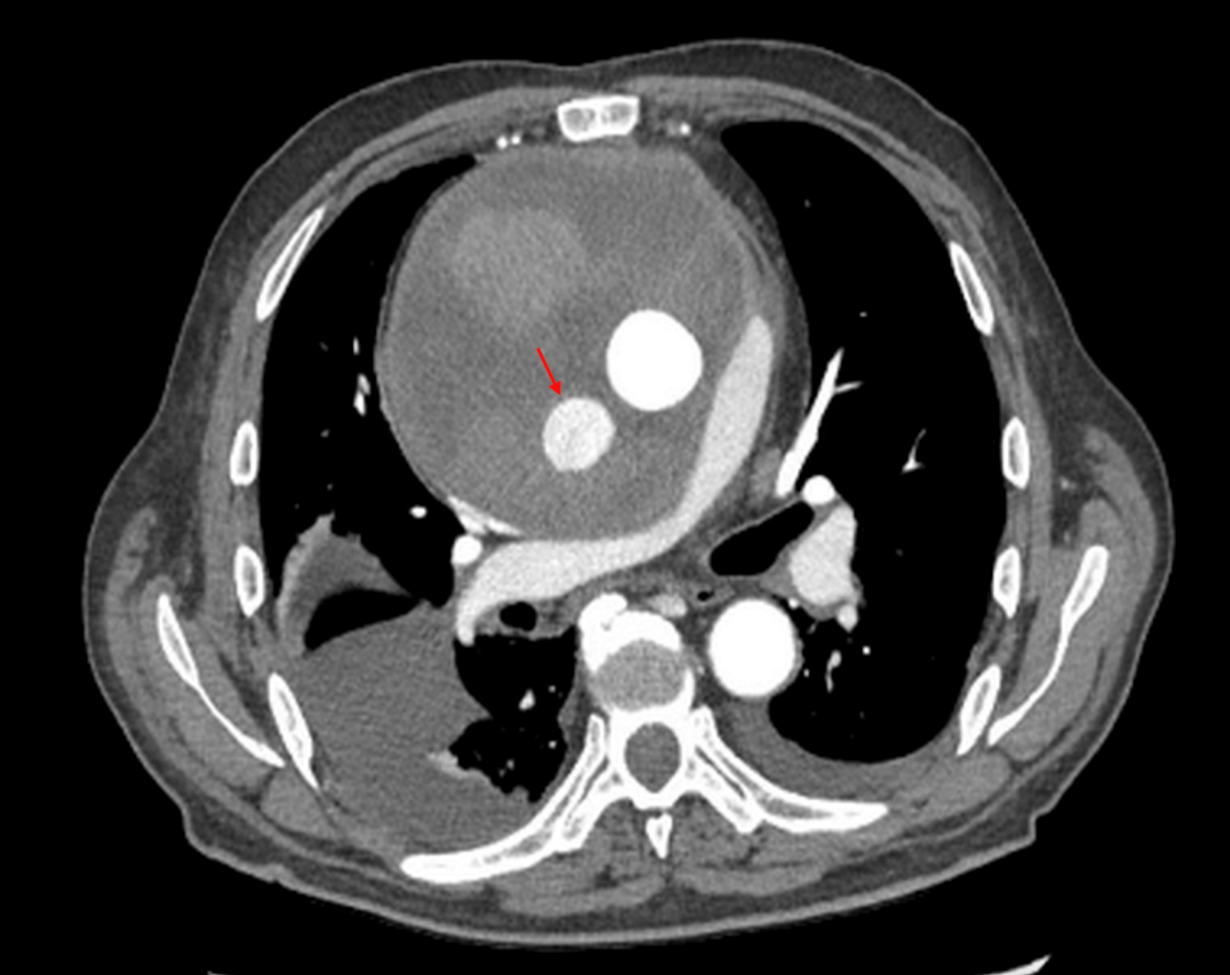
Repeat CT aorta three days after presentation A 3 cm enhancing pseudoaneurysm arising from the posterolateral aspect of the ascending aorta (red arrow), with an increase in the size of the hematoma by 0.5 cm in each dimension.
